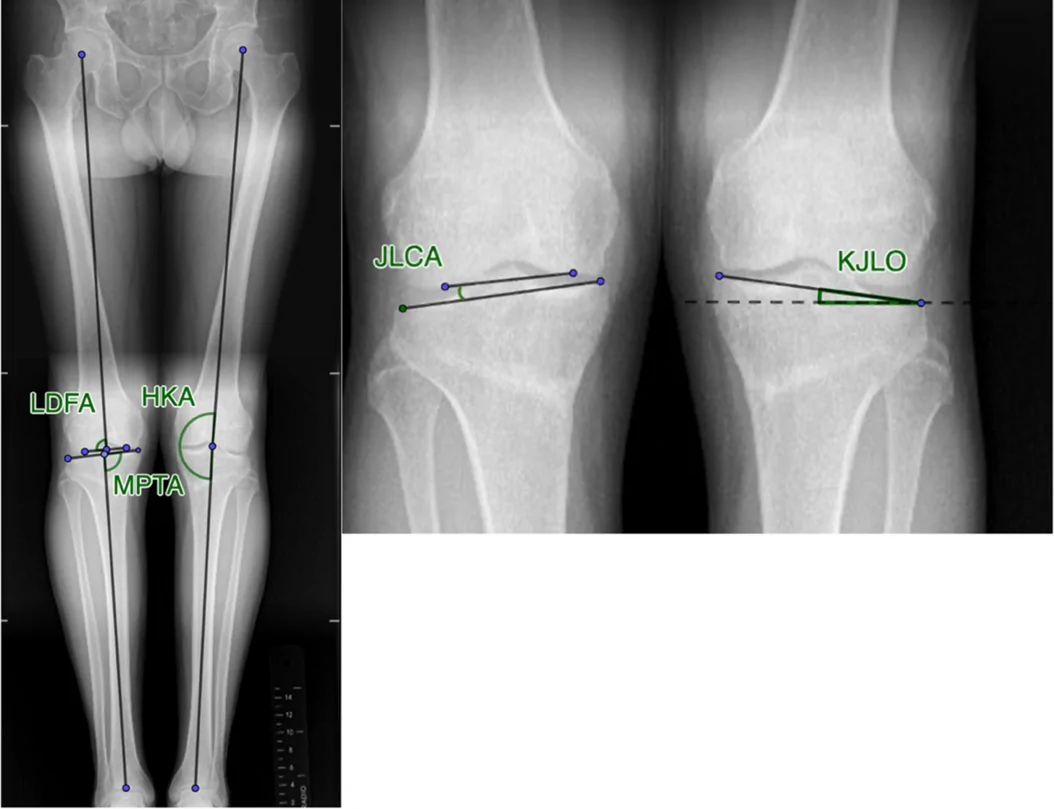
Radiographic measurements. HKA, hip–knee–ankle angle; JLCA, joint line convergence angle; KJLO, knee joint line obliquity; LDFA, lateral distal femoral angle; MPTA, medial proximal tibial angle.
